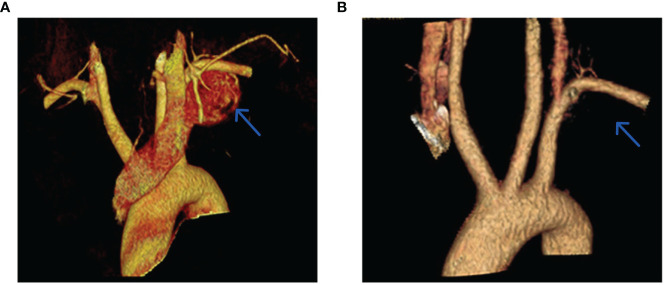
Three-dimensional reconstruction CT scans of tumor vessels were obtained preoperatively (A) and postoperatively (B).
